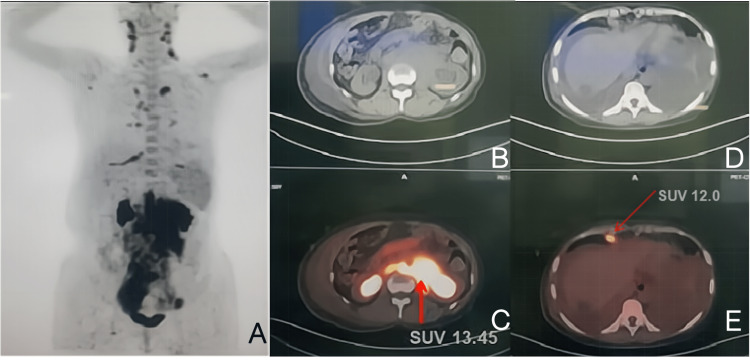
PET-CT of December 2018. The soft tissue with FDG hypermetabolism located in the nearby abdominal aorta, vena cava, and small intestine mesenteric and presacral area (A-E). The SUVmax of it was 13.45 (C). PET: positron emission tomography; FDG: fluorodeoxyglucose; SUVmax: maximum standardized uptake value
